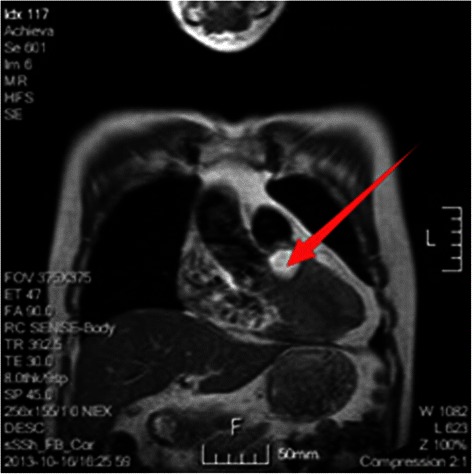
Cardiac MRI showed a round-like signal, measuring 36 × 20 mm, in the upper portion of IVS, anterior to the right coronary sinus of aorta
